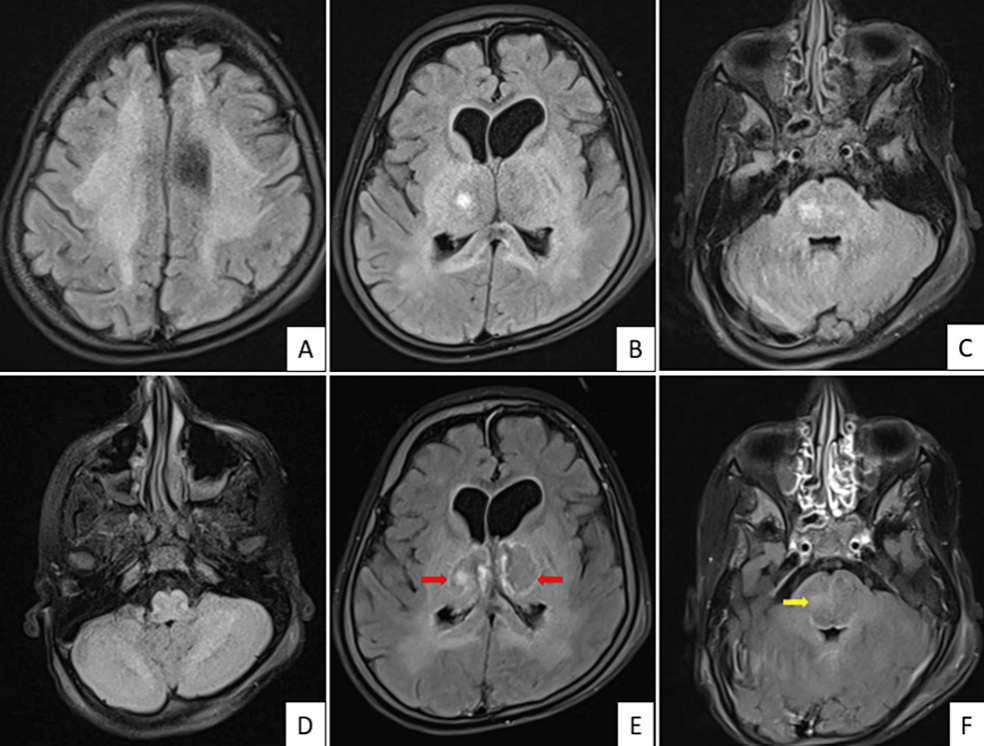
The FLAIR images (A–D) show bilateral diffuse symmetric hyperintense signal in the centrum semiovale (A), thalamus (B), pons (C), and cerebellum (D). Post-contrast FLAIR images (E, F) show peripheral contrast enhancement in the bilateral thalamus (red arrows) and subtle enhancement in the pons (yellow arrow). FLAIR: fluid-attenuated inversion recovery
